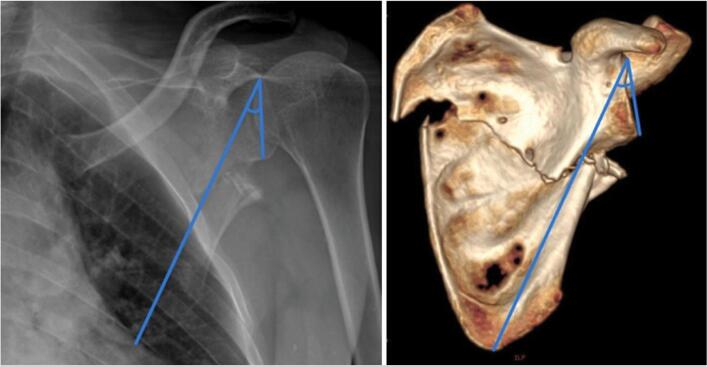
Measurement of the glenopolar angle in an AP radiograph taken in the emergency room and in a CT-3D scan of the same patient.
